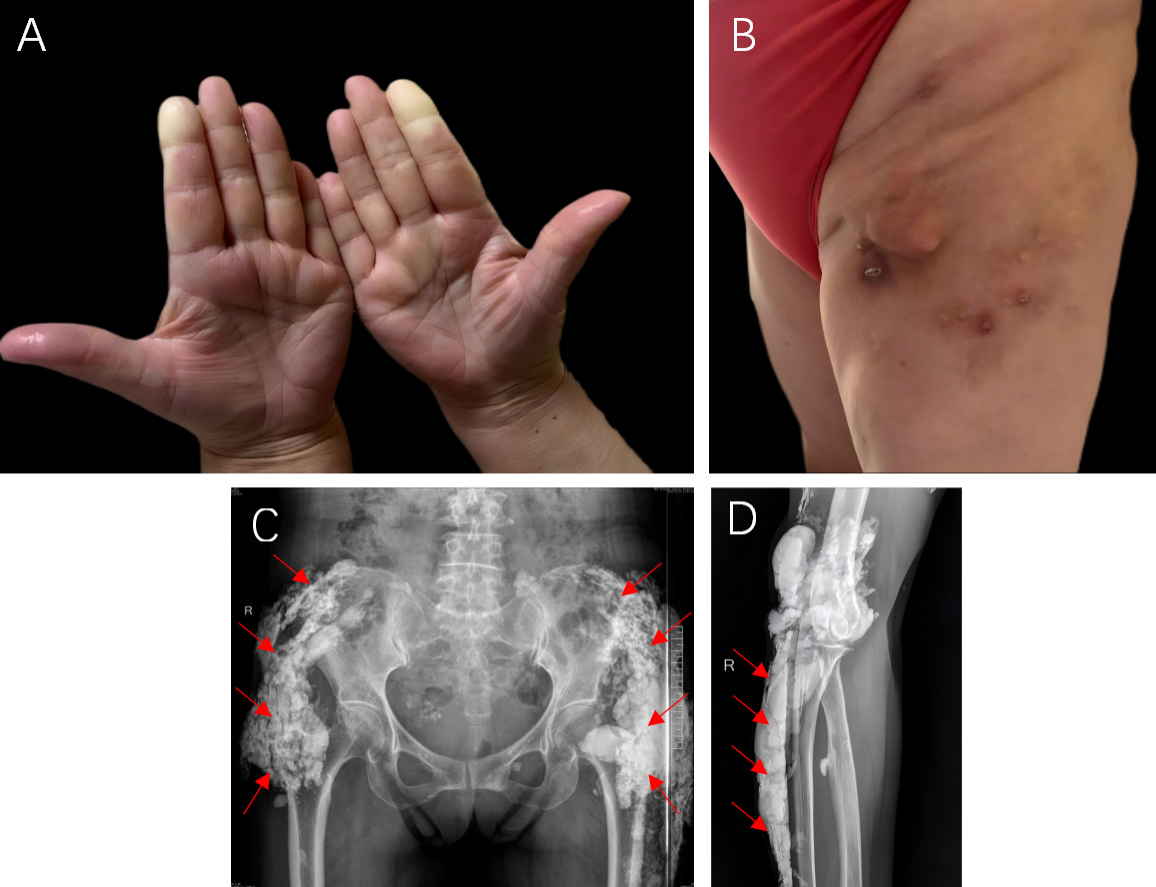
Clinical manifestations and imaging findings. A. Raynaud phenomenon. B. Multiple subcutaneous firm nodules on the extremities, which had ulcerated and released white, calcium-like material. C & D. X-rays of the upper extremities, pelvis, and femur showing multiple patchy calcifications in the pelvis, femur, and adjacent soft tissues.
